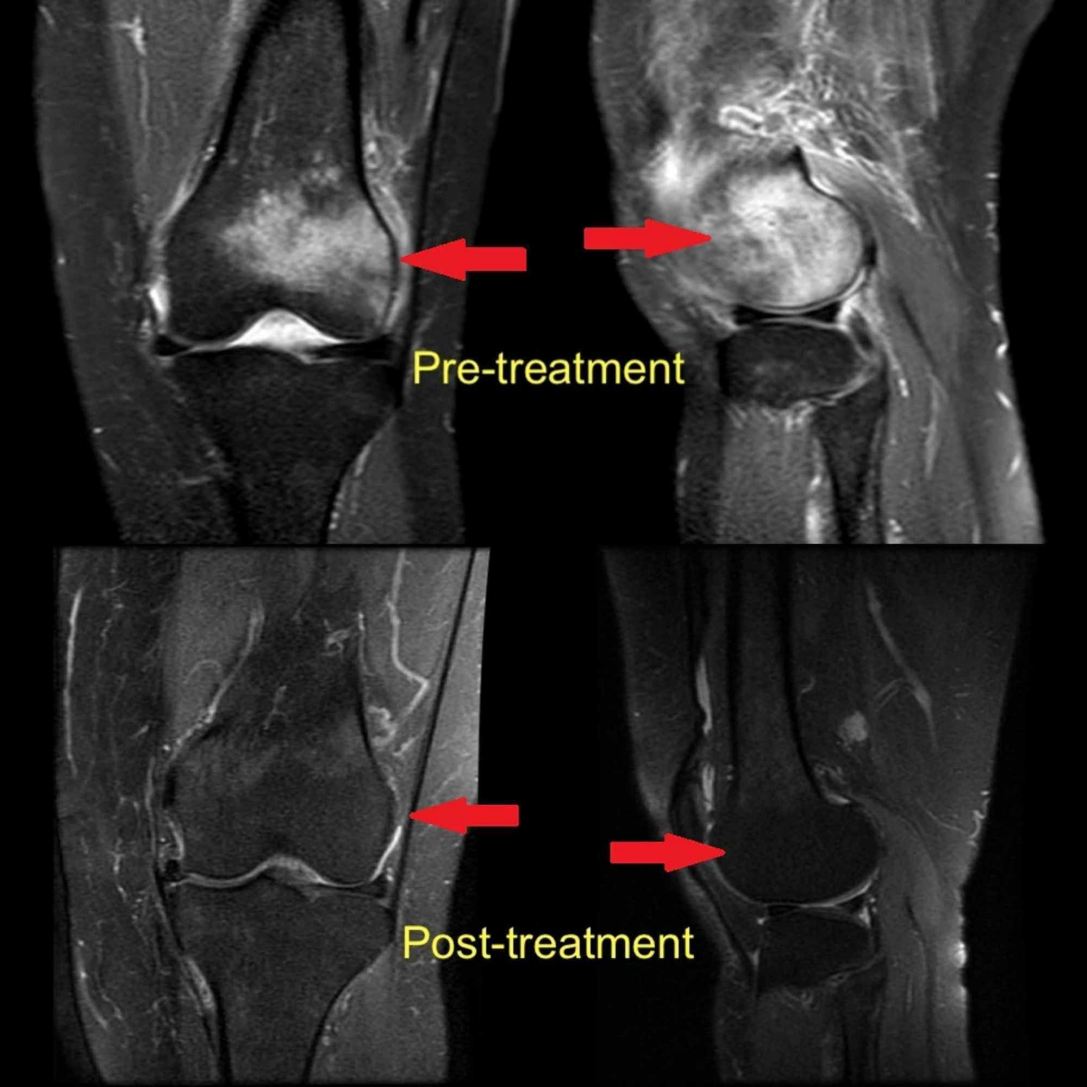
MRI appearance of bone marrow edema of the lateral femoral condyle (arrows) MRI: magnetic resonance imaging
